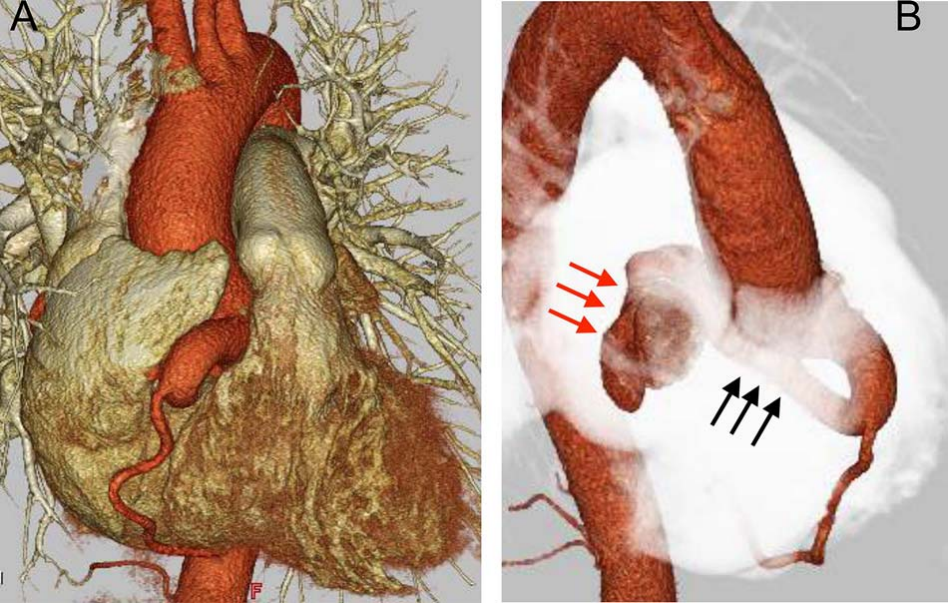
Preoperative CT findings; (A) frontal view; (B) upper right diagonal view; preoperation CT showed a dilated RCA (black arrow) with a diameter of 13.2 mm, and a giant aneurysm (red arrow) with an RCA-to-right atrium fistula.
